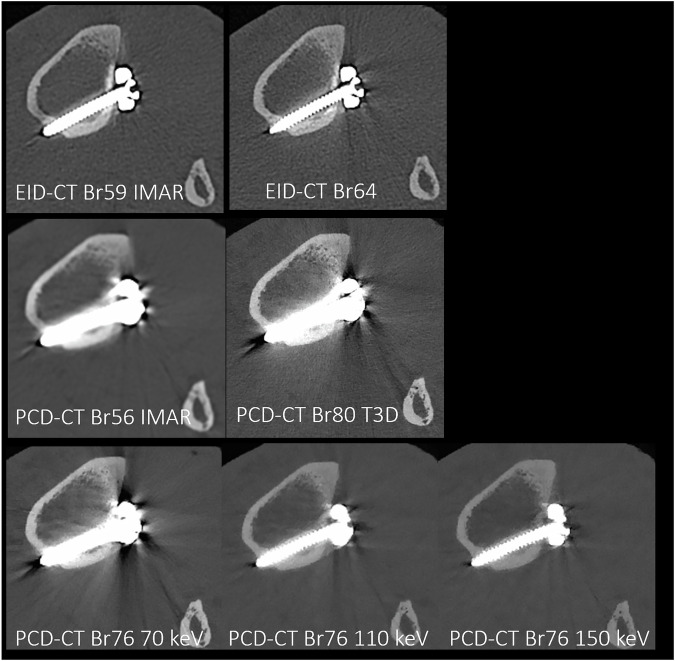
Representative axial CT images showing the metal-bone interface for a screw in the tibia. The top row displays EID-CT images, while the middle and bottom rows show PCD-CT images
